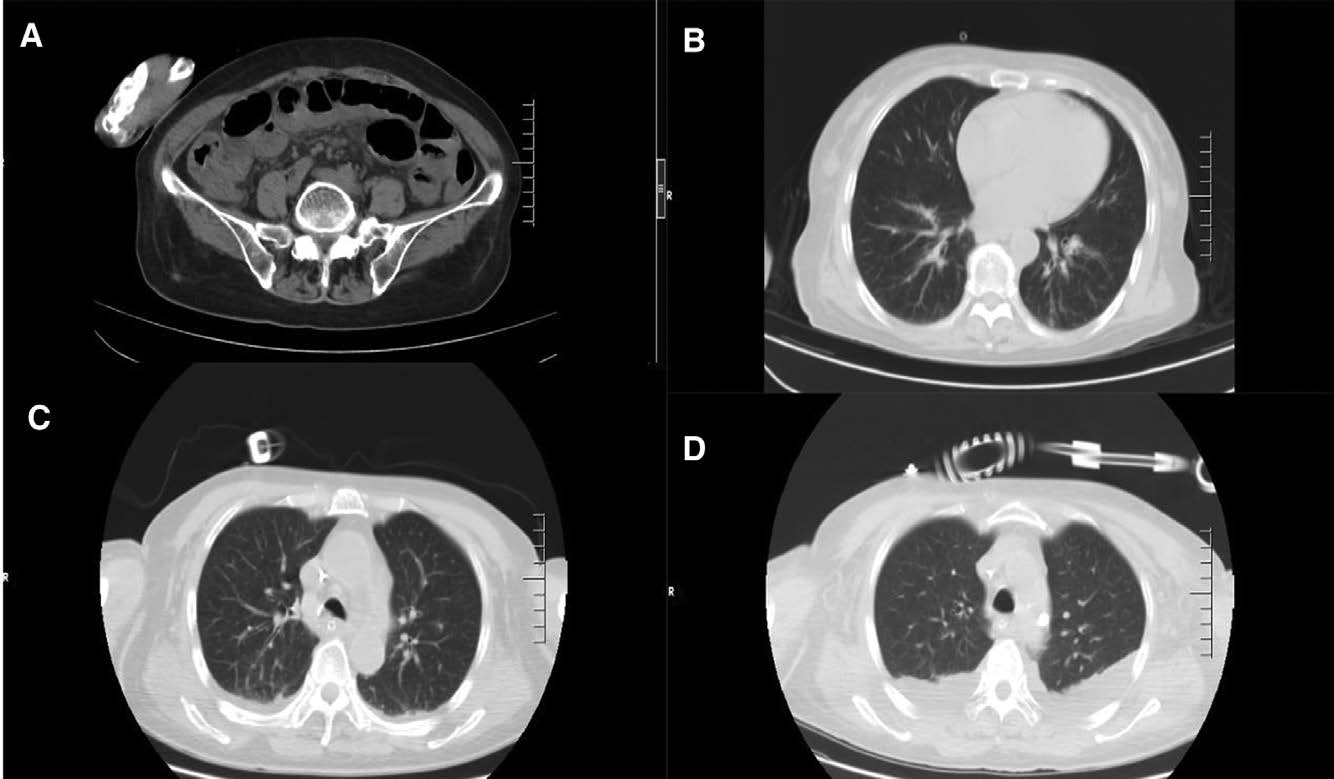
CT of the chest and abdomen since admission. (A) Abdominal CT on July 11 showed that part of the small intestine was dilated, fluid accumulation, gas accumulation, and gastric cavity was dilated. (B) Chest CT on July 11 showed no obvious inflammation and other abnormalities in the lungs. (C) Chest CT on July 15 showed a small bilateral pleural effusion. (D) Chest CT on July 19 showed partial external pressure atelectasis with bilateral pleural effusion. CT = computed tomography.
